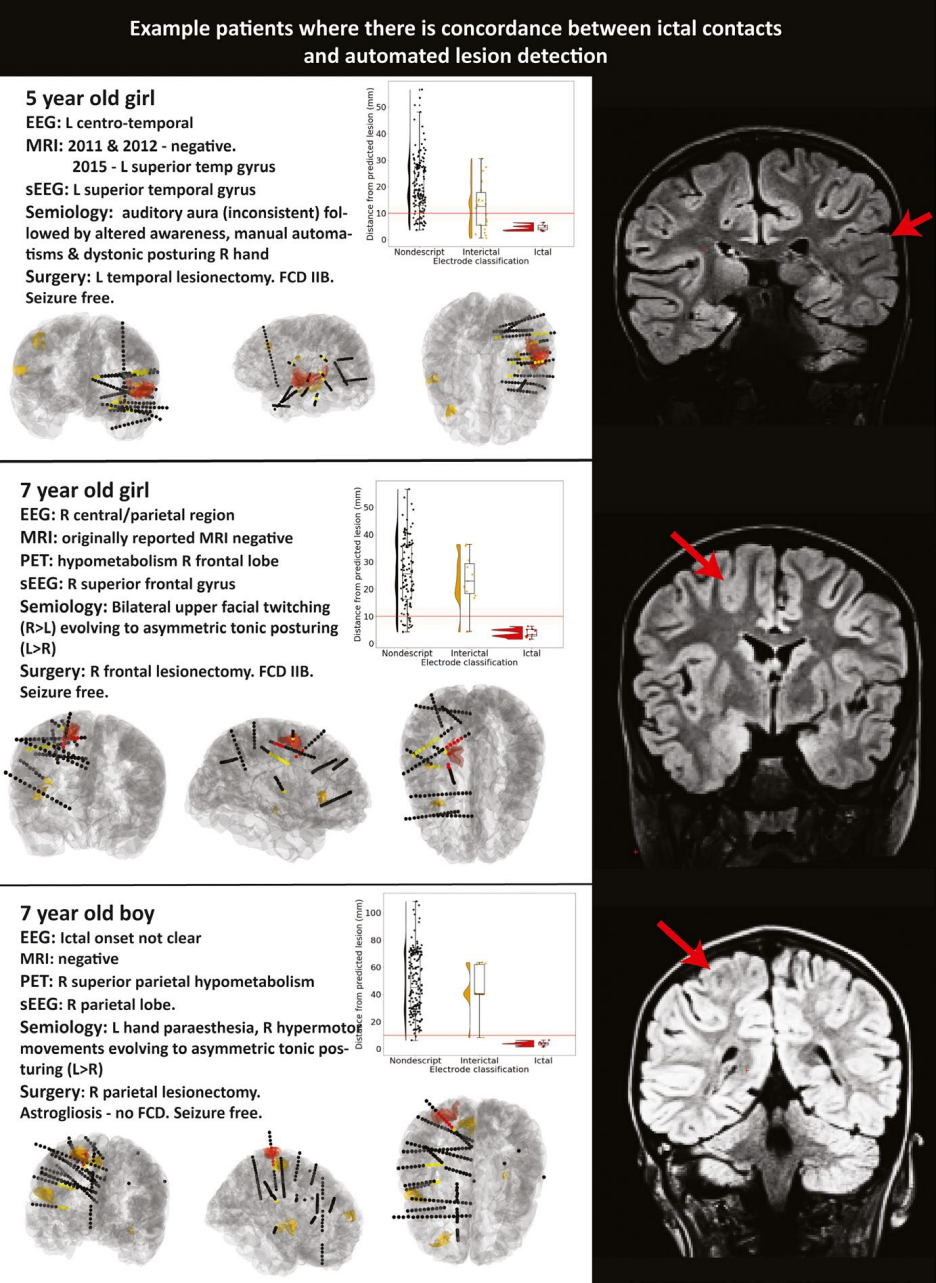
Case studies of three sample patients where there is colocalization between ictal contacts and automated lesion detection. Ictal contacts (red contacts) are within 10 mm of the automated classifier prediction (red cluster). For each patient, a brief clinical overview (left upper), a plot of distance of the stereoelectroencephalography (sEEG) contacts from the predicted lesion (right upper), visualization of the electrode positioning (ictal contacts = red, interictal = yellow, other = black) with automated clusters (red = top cluster, yellow = other clusters, lower panels), and a coronal section of fluid‐attenuated inversion recovery magnetic resonance imaging (MRI) with lesion indicated by red arrow are shown. L, left; R, right; FCD, focal cortical dysplasia; PET, positron emission tomography
